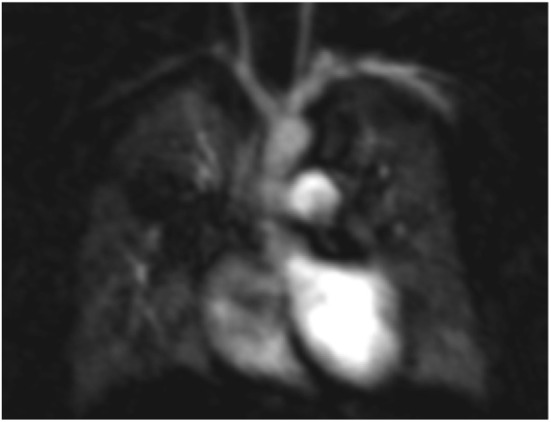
Magnetic resonance imaging angiogram in a child with bronchopulmonary
